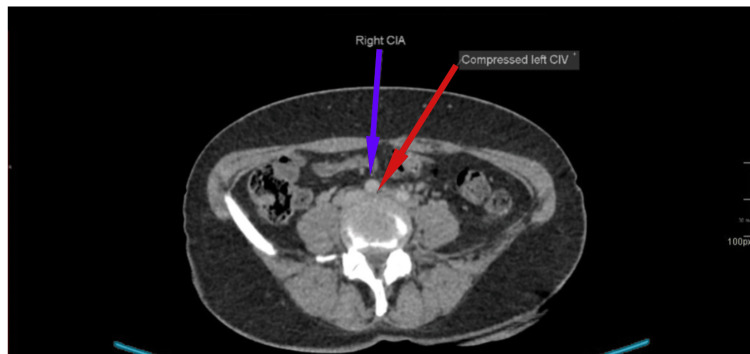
Compressed left common iliac vein (CIV) with right common iliac artery (CIA) in front on CT thorax, abdomen, and pelvis (CT TAP) CT: computed tomography
